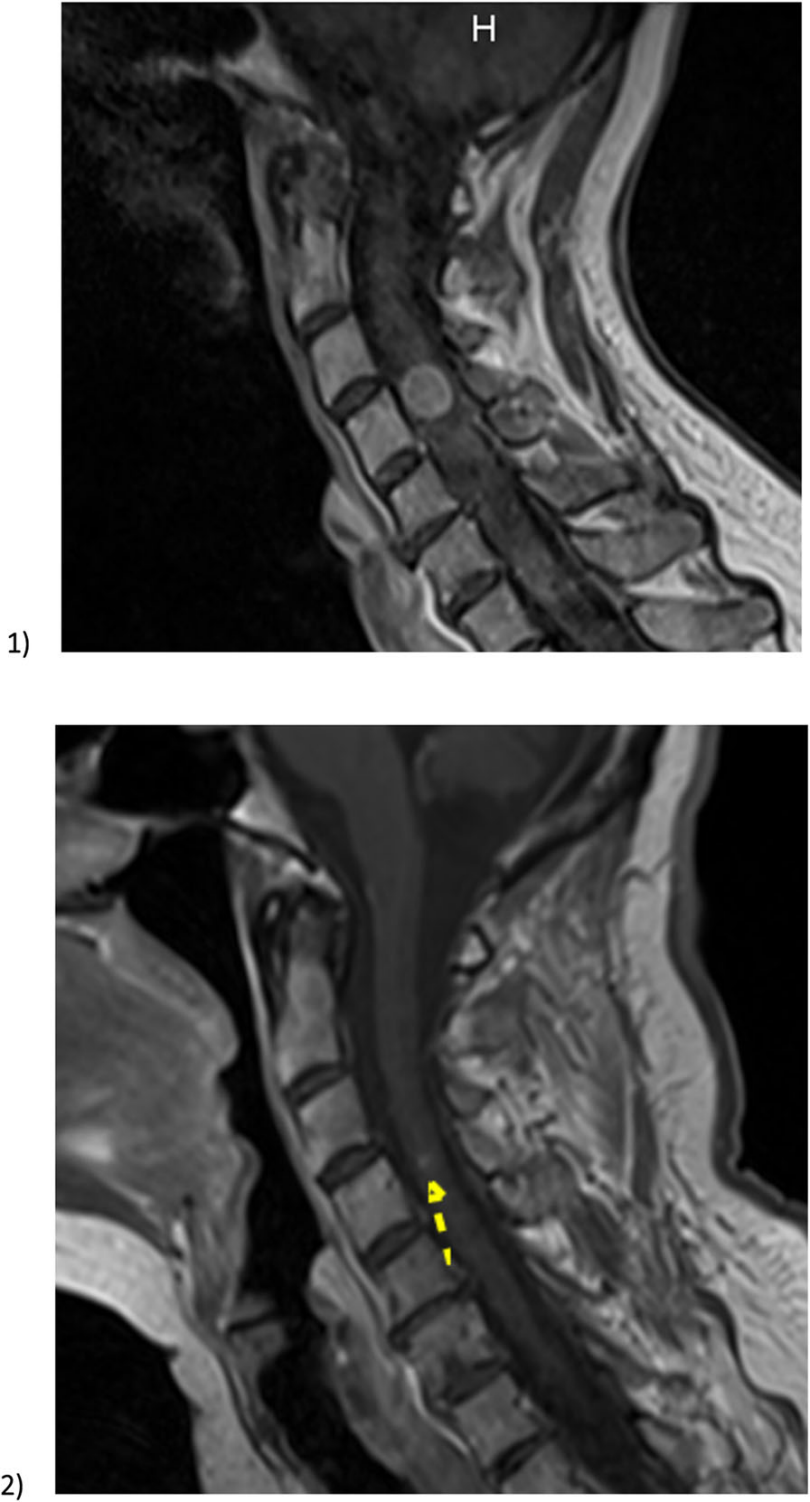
Magnetic resonance imaging (MRI) of patient 5 who was treated with a dose of 30 Gy in five fractions. 1) Pre-stereotactic body radiotherapy (SBRT) MRI with metastasis opposite C4. 2) MRI at 3 months after completing radiotherapy with almost complete response observed
